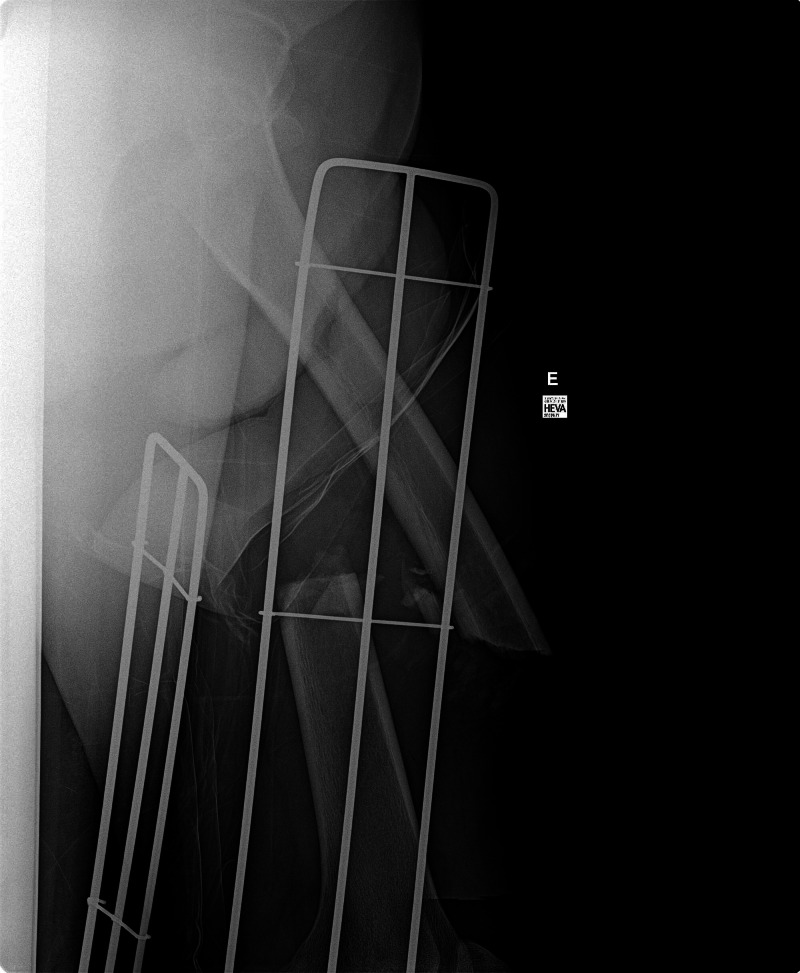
X-ray showing open displaced femur fracture Case courtesy of Prof. Cláudio Souza, Radiopaedia.org, rID: 13772.
